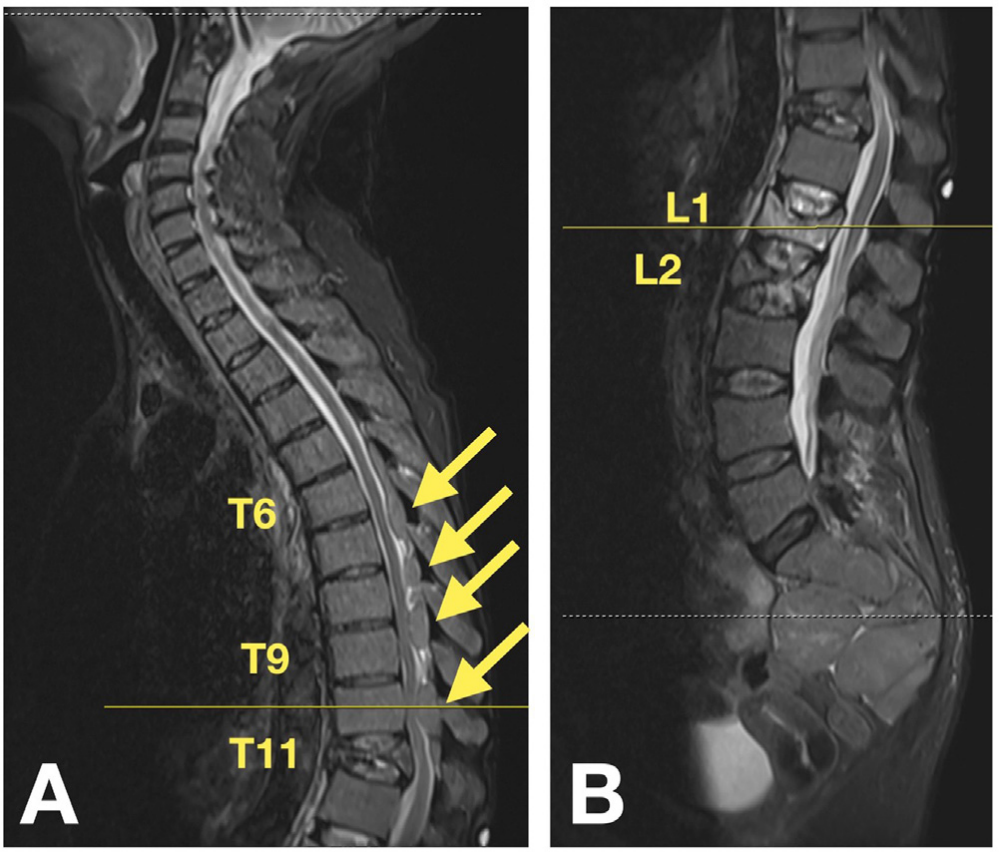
Initial thoracic (A) and lumbar (B) MRI demonstrating compression fractures at T11, L1, and L2 and the dorsal extradural masses from T6 to T10.
